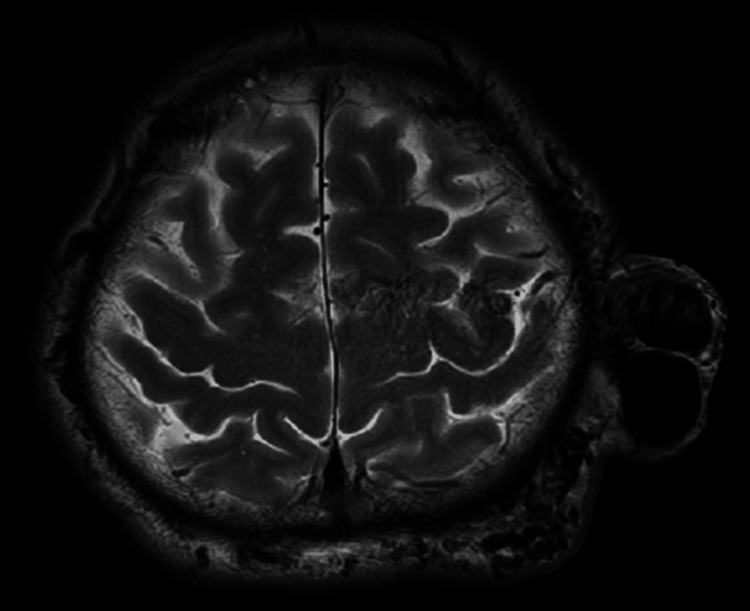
Initial head MRI T2-weighted MRI reveals a vascular tumor-like lesion in the subcutaneous tissue, measuring up to 80 mm in maximum diameter. MRI: magnetic resonance imaging
